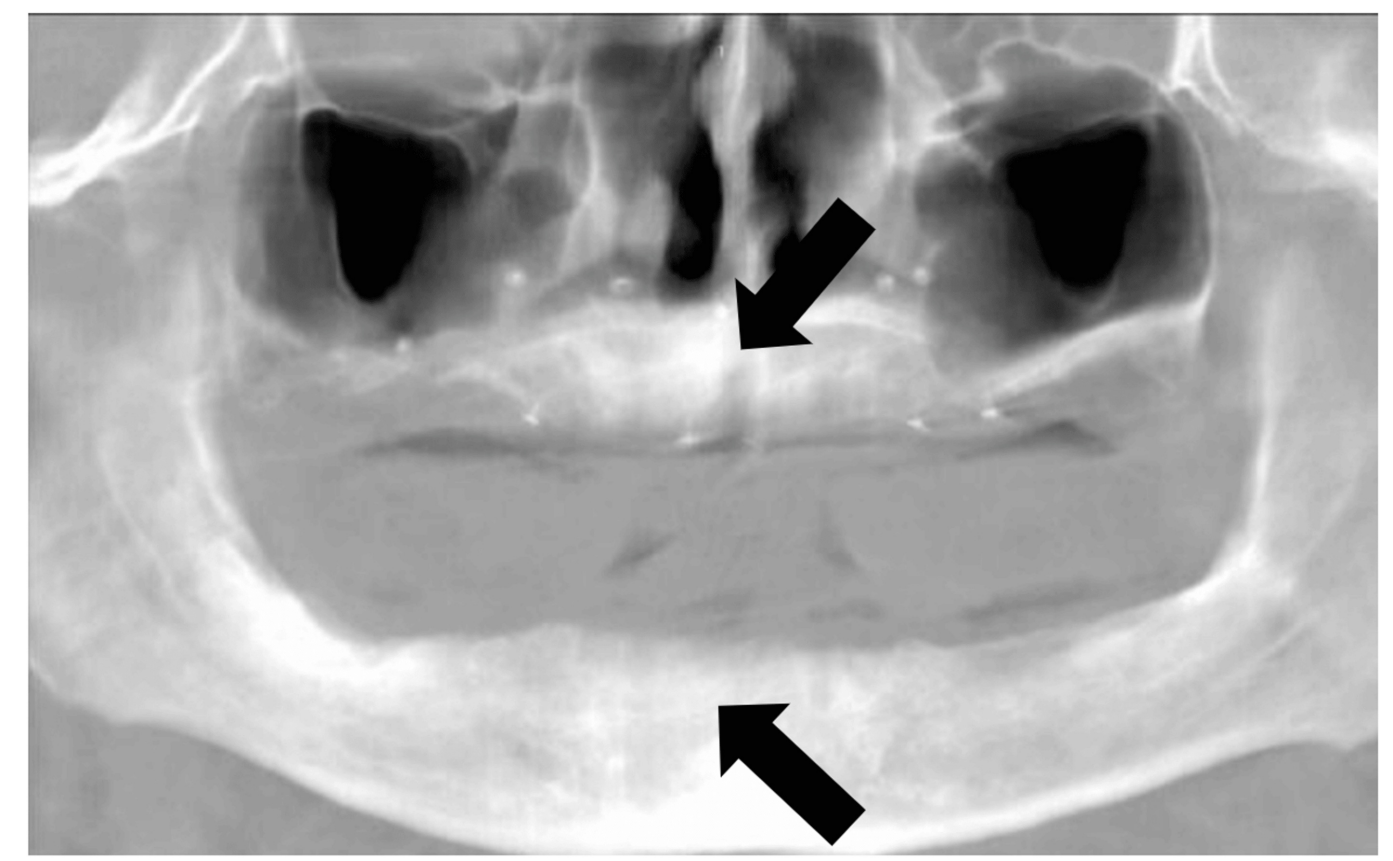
Tomographic image at 12 months. The arrows indicate areas of dimensional stability and minimal change as confirmed by standardized CBCT measurements and image superimposition.
